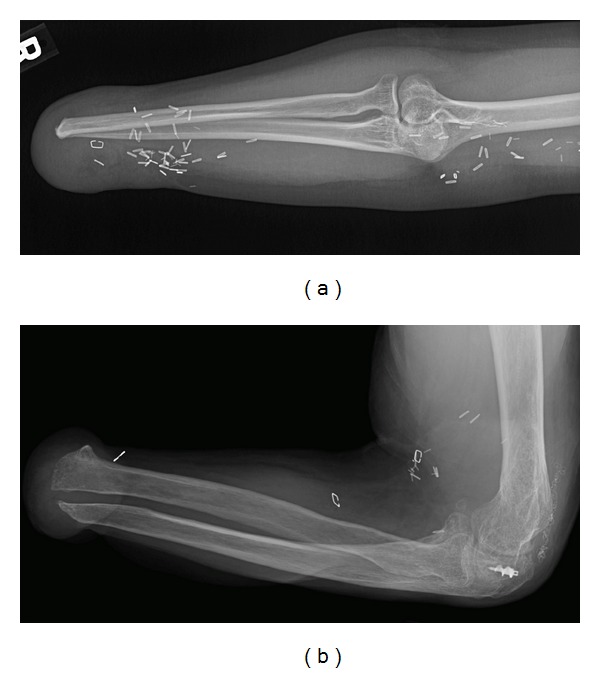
(a) Radiograph of selected candidate shows osseous integrity of the amputation site and no significant arthropathy of the proximal joint. (b) Radiograph showing diffuse osteopenia and proximal arthropathy of a patient who failed screening.
